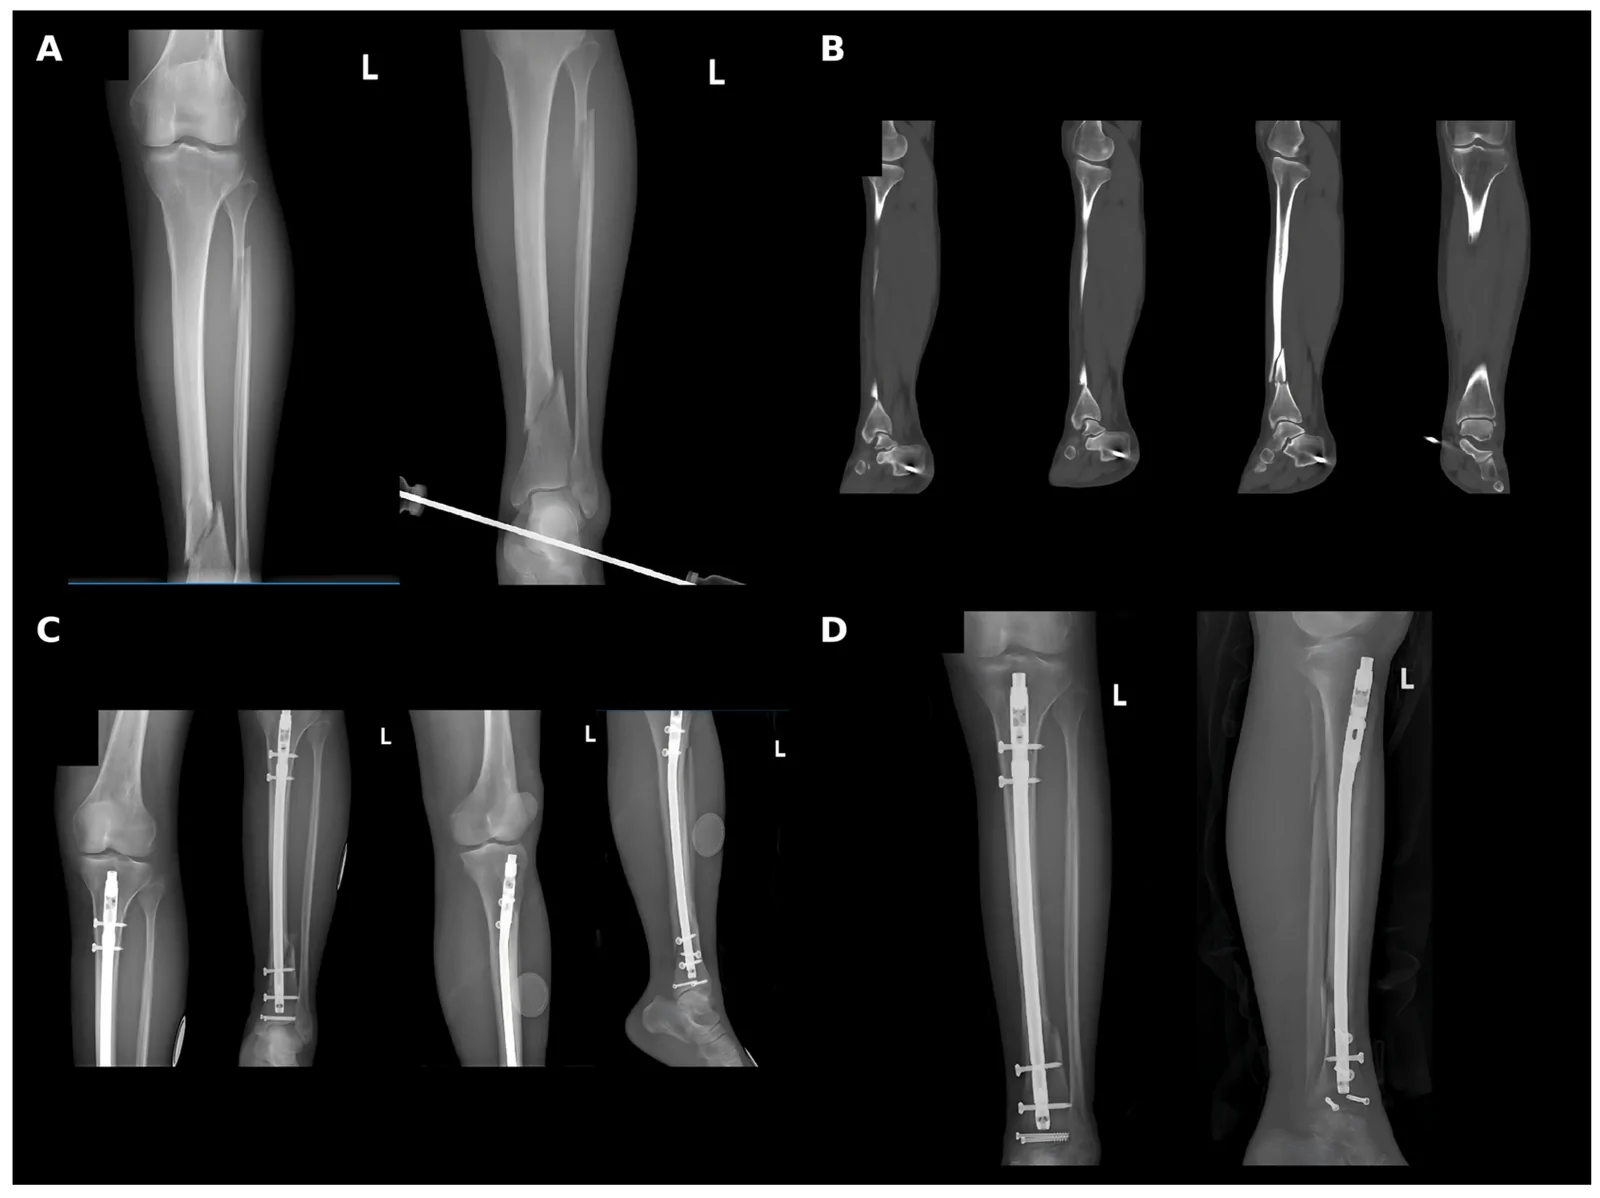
Representative case treated with intramedullary nailing. (A) Preoperative anteroposterior and lateral radiographs showing a distal tibial fracture; (B) preoperative computed-tomography images demonstrating fracture morphology; (C) postoperative anteroposterior and lateral radiographs after locked antegrade intramedullary nailing with distal interlocking; (D) follow-up radiographs showing maintained implant position and alignment with interval fracture healing. L, left side.
